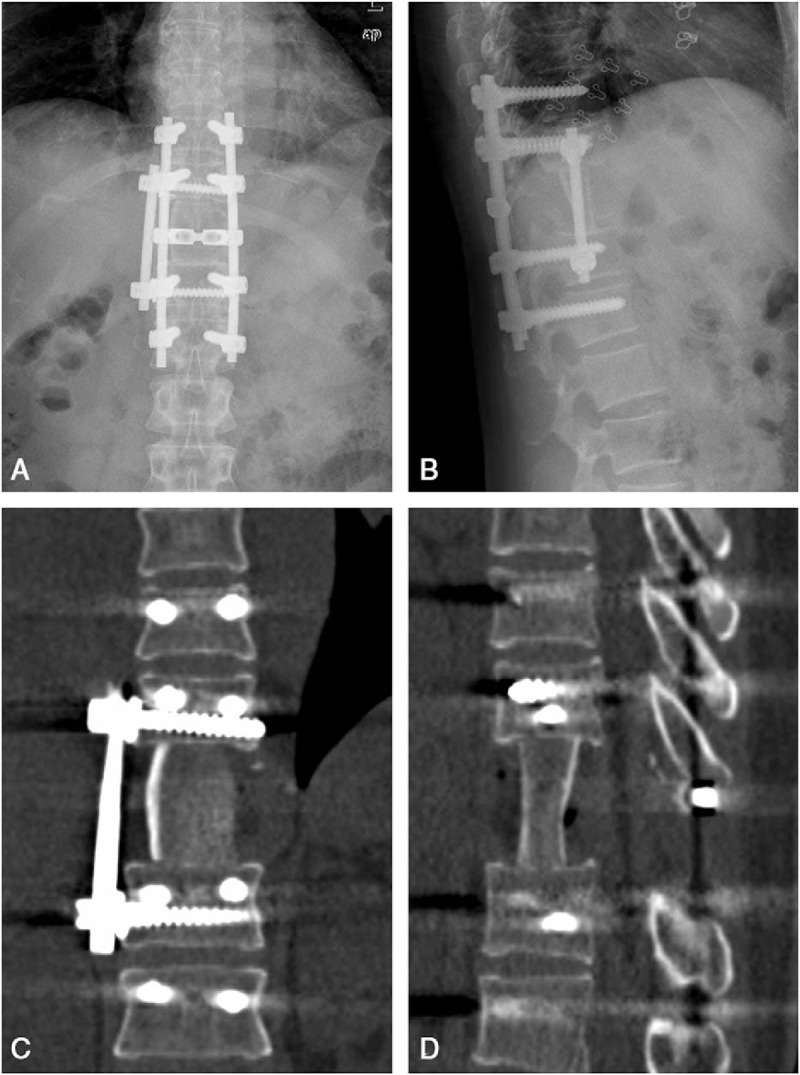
Postoperative immediate x-ray and 3-dimensional computed tomography (CT) scan images. (A) Anteroposterior x-ray; (B) Lateral x-ray; (C) coronal CT; (D) Sagittal CT.
